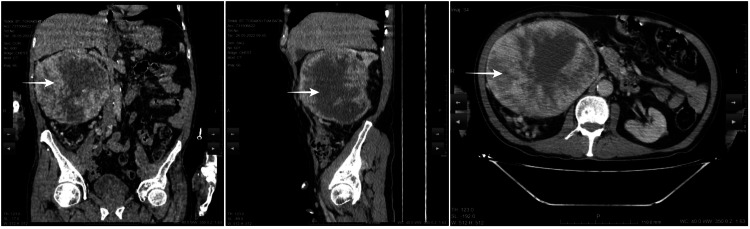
Contrast-enhanced computed tomography images Arrow indicates mass in the antero-mid-lower part of the right kidney with an axial diameter of 167×146 mm, a heterogeneous mass of soft tissue density with central necrosis, consistent with RCC.
